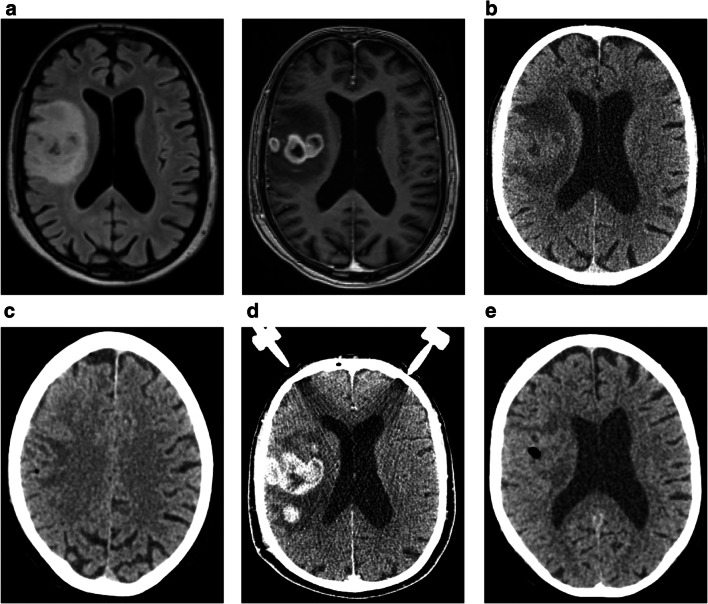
Patient was admitted to neurosurgery with FLAIR hyperintense lesion (a, left panel) with partial ring enhancement and perifocal edema (a, right panel) highly suggestive for high-grade brain tumor. Biopsy planning included CT-based navigation (b). CT after first biopsy reveals small air bubble representing sample location mostly within perifocal edema (c). Since first biopsy result was non-conclusive, re-biopsy was planned using contrast-enhanced CT with stereotactic frame (d). CT after second biopsy shows a more central sampling as suggested by an according air bubble (e). Second biopsy revealed glioblastoma as diagnosis
